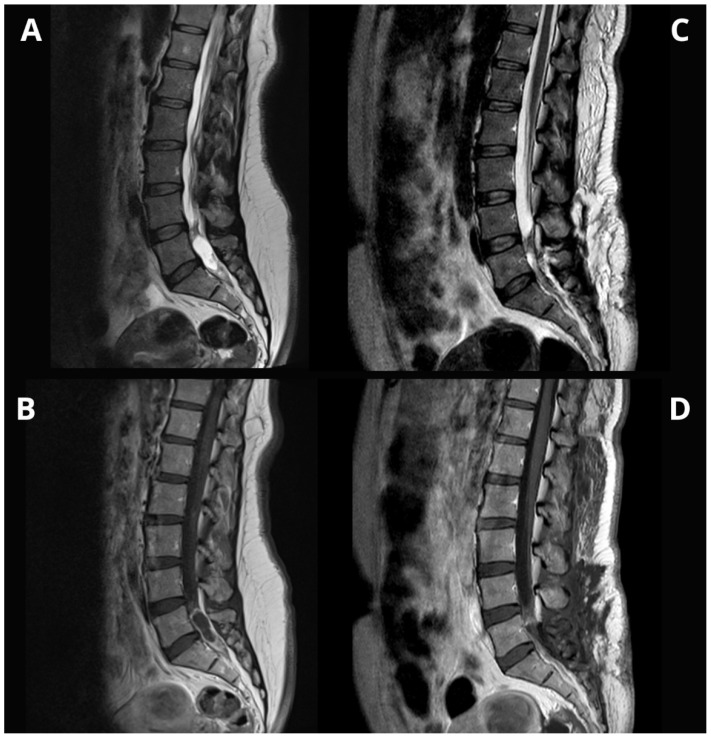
Pre- and postoperative imaging comparison of a dysembryoplastic neuroepithelial tumor (DNET) disseminated lesion in the lumbosacral region. (A) Preoperative T1-weighted MRI showing the DNET secondary lesion at the mid-L5 level, extending downward into the sacral canal with peripheral enhancement. (B) Postoperative T1-weighted MRI following L5 laminectomy, demonstrating complete resection of the spinal lesion with no residual tumor. (C) Preoperative STIR MRI highlighting the lesion’s extent and peripheral enhancement. (D) Postoperative STIR MRI confirming successful resection with no evidence of residual lesion or abnormal signal intensity.
